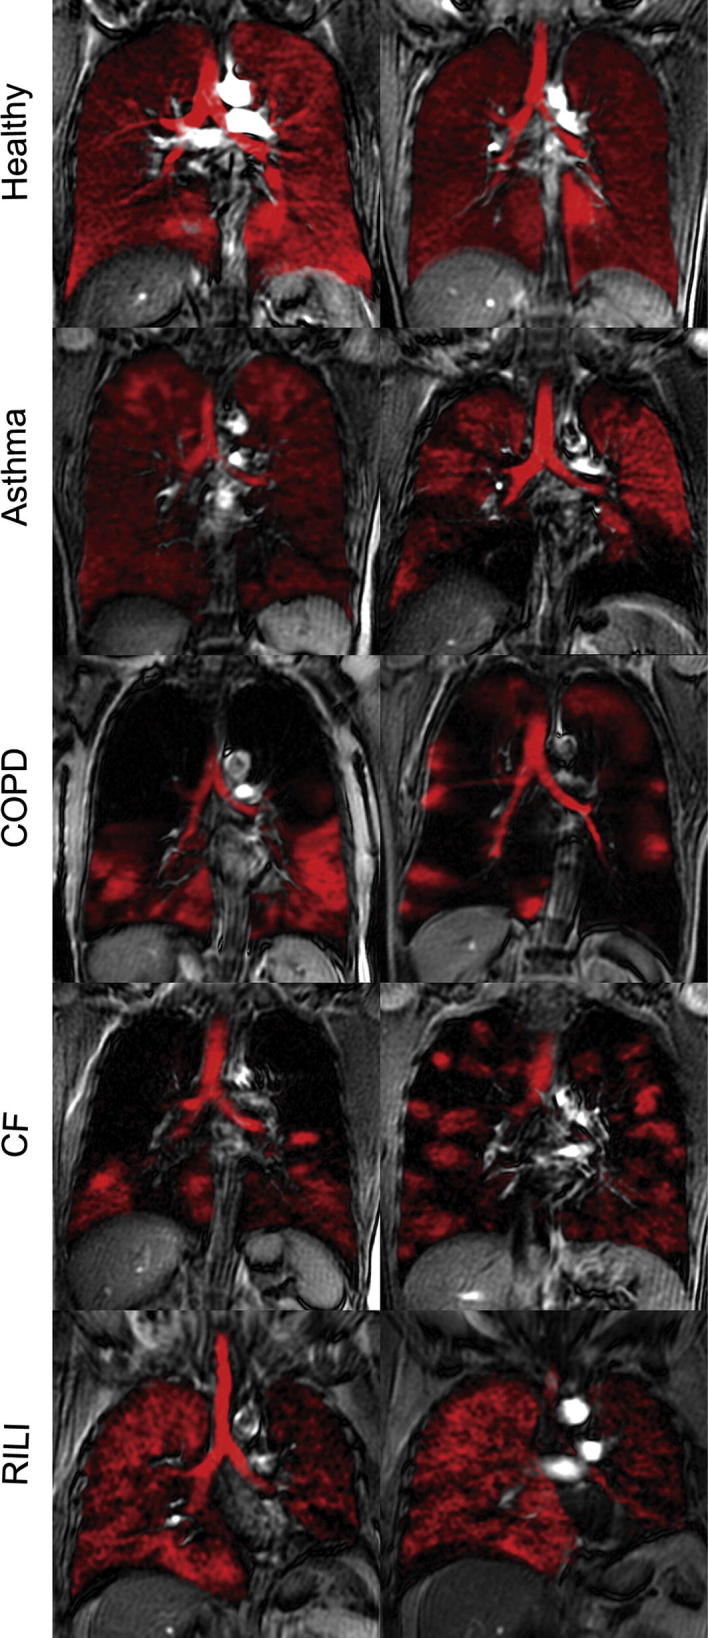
Hp ^129^Xe slice selective coronal MR images (in red) overlayed onto corresponding proton thoracic images from healthy volunteers and subjects with asthma, chronic obstructive pulmonary disease (COPD), cystic fibrosis (CF) and radiation-induced lung injury (RILI). Reprinted with permission from Shukla et al. Acad. Radiol., 2012; 19:944, © 2012 Elsevier. (For interpretation of the references to color in this figure legend, the reader is referred to the web version of this article.)
